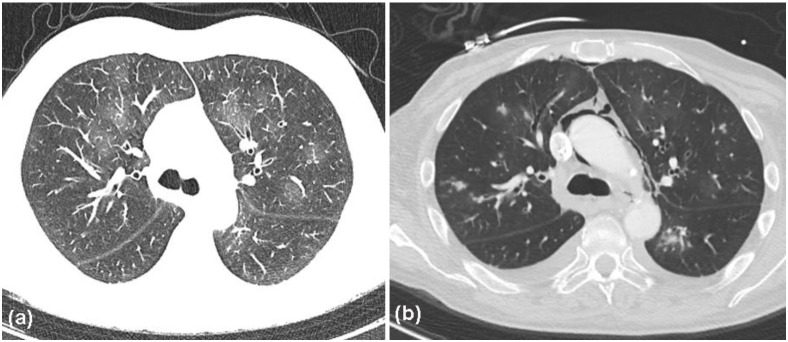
Computed tomography (CT) scans of the chest. (a) Subtle mosaic attenuation, ground-glass opacities, bronchiectases, and bronchial wall thickening, consistent with a diagnosis of bronchiolitis obliterans (BO); (b) pneumomediastinum as a further complication of BO.
